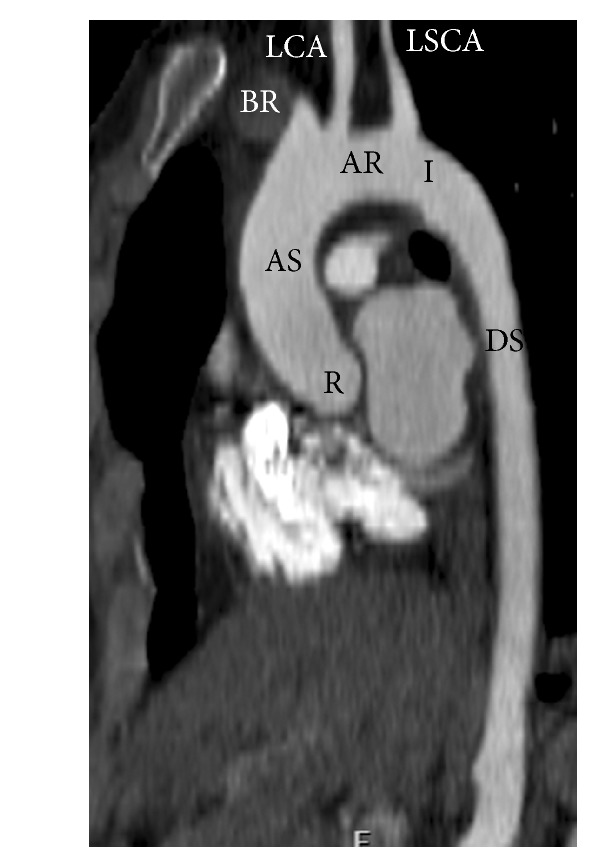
Normal  thoracic  aorta—sagittal reconstructed CT image of thoracic aorta showing the various segments of thoracic aorta and the arch branch vessels. R: root; AS: ascending aorta; AR: arch; I: isthmus; DS: descending thoracic aorta. BR: brachiocephalic artery; LCA: left common carotid artery; LSCA: left subclavian artery.
